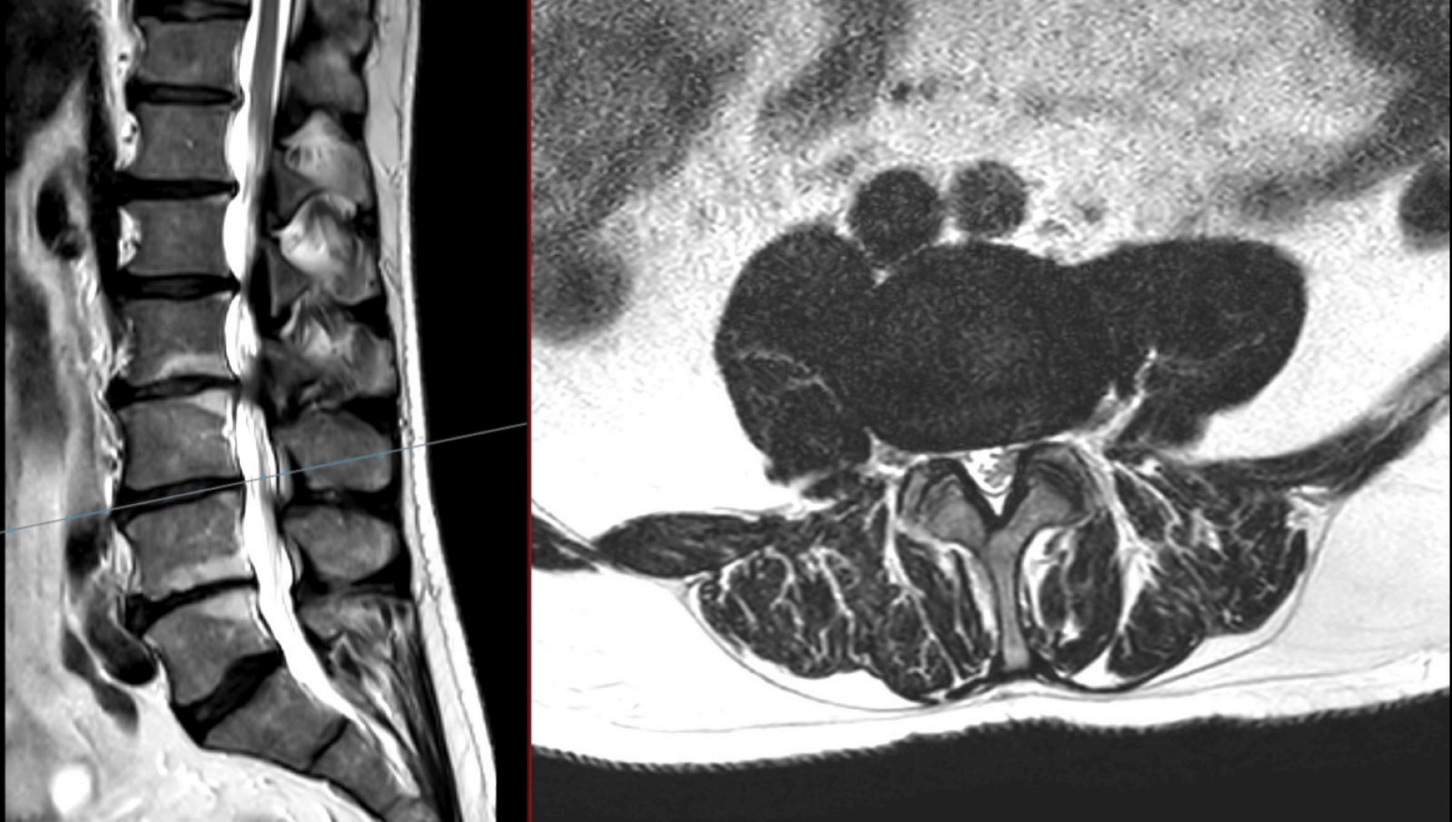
T2 MRI of the lumbar spine: sagittal (left), axial (right) at L3/4 No evidence of spinal stenosis and compression of the cauda equina was noted.
